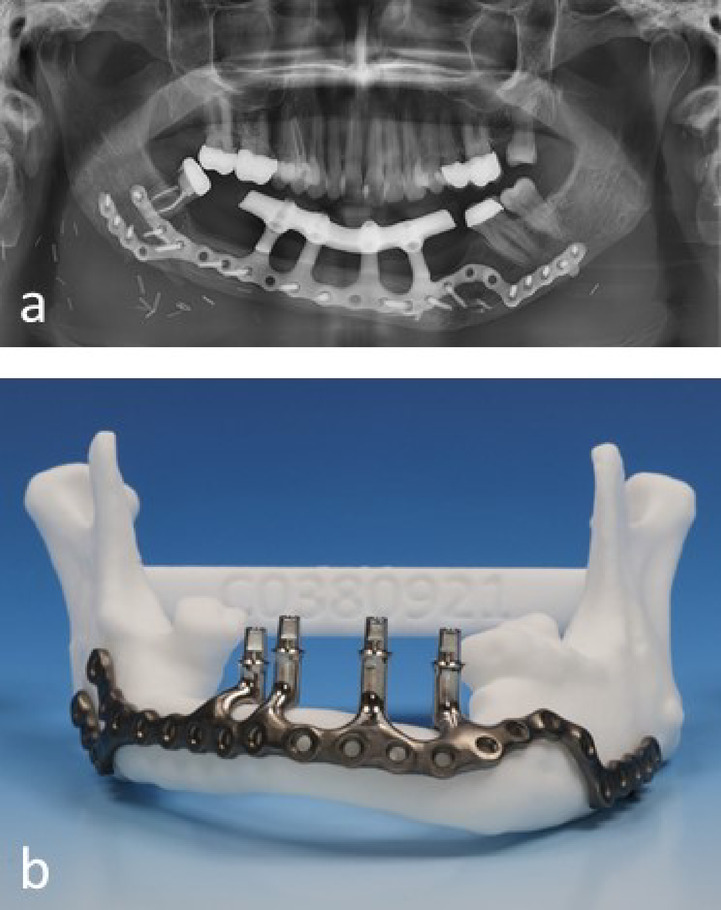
(a) Orthopantomograph with an IPS Implant in the patients lower jaw. An individual bar is screwed onto the coupling elements to anchor a removable denture. (b) IPS Implant of the patient shown in Figs. 1a and 2 on a 3D-printed model.
